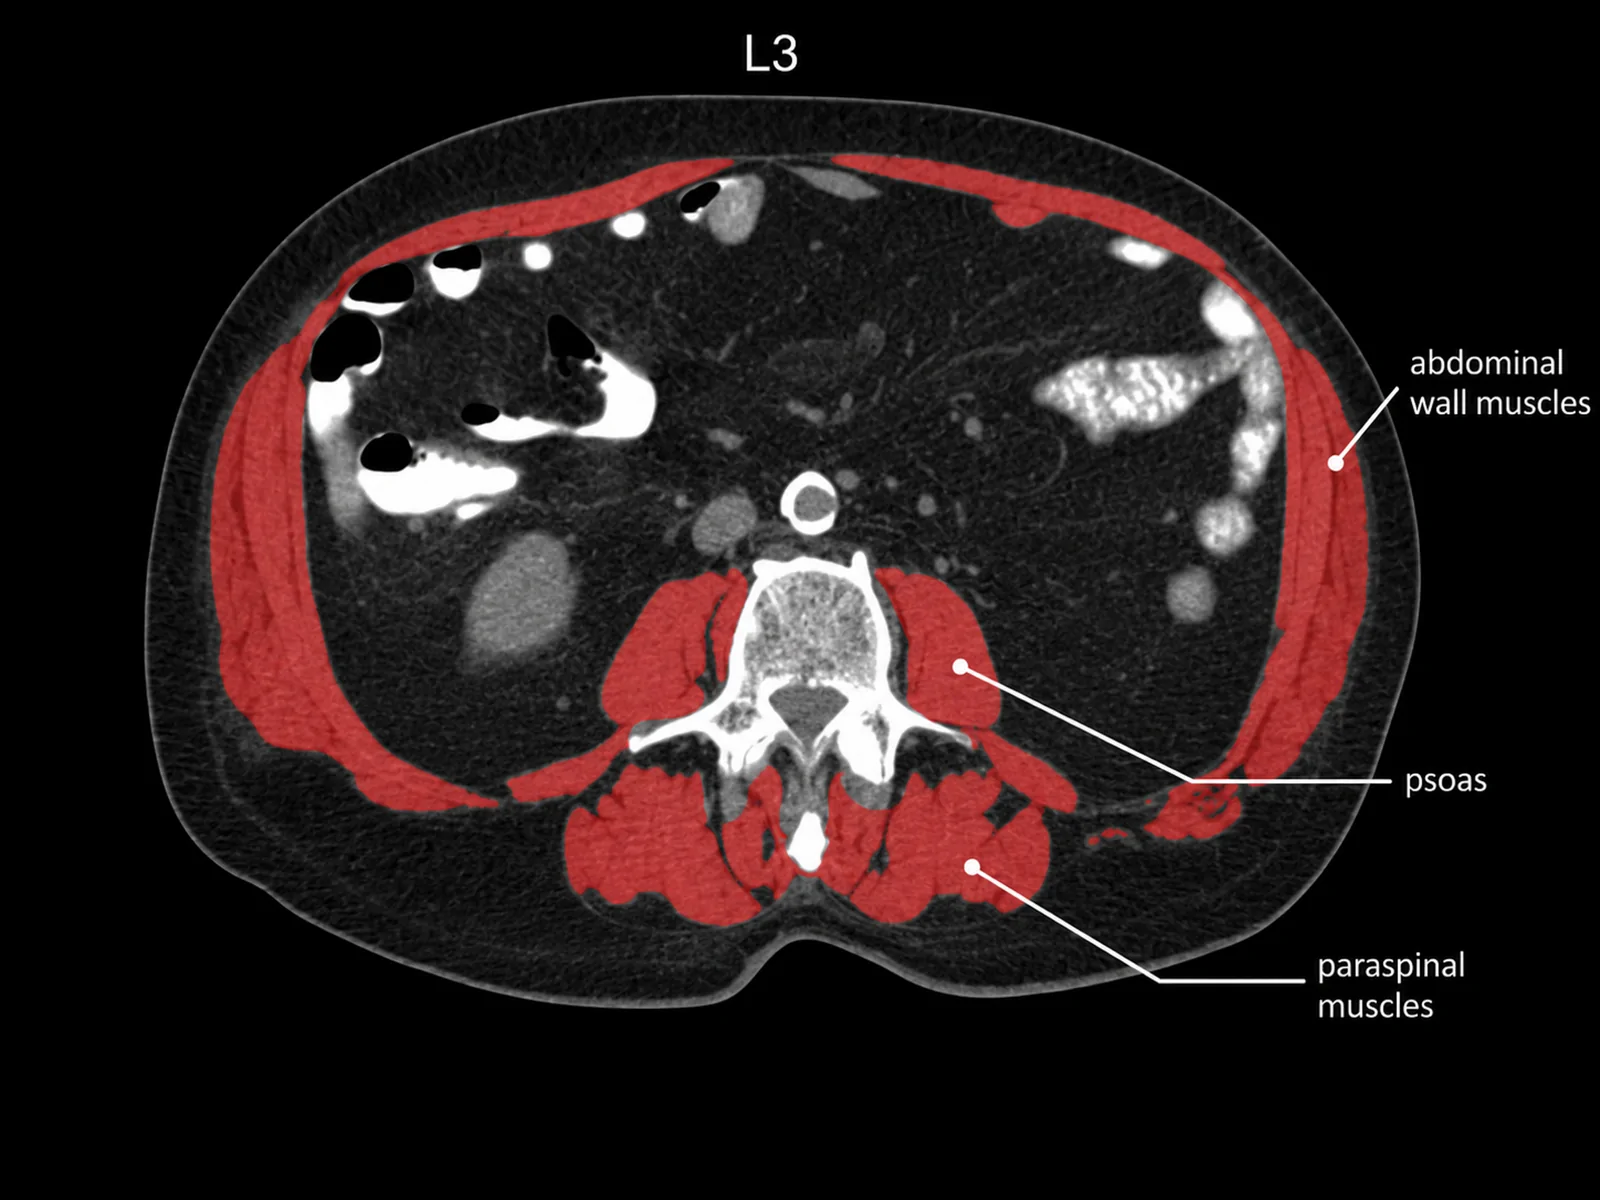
Representative L3 skeletal muscle segmentation on axial CT. Axial CT image at the level of the third lumbar vertebra (L3) showing segmentation of the skeletal muscle area used for skeletal muscle index (SMI) assessment. The highlighted regions include the psoas, paraspinal, and abdominal wall muscles. Skeletal muscle area was measured using predefined Hounsfield unit thresholds (− 29 to + 150 HU) and normalized for height squared to calculate SMI
